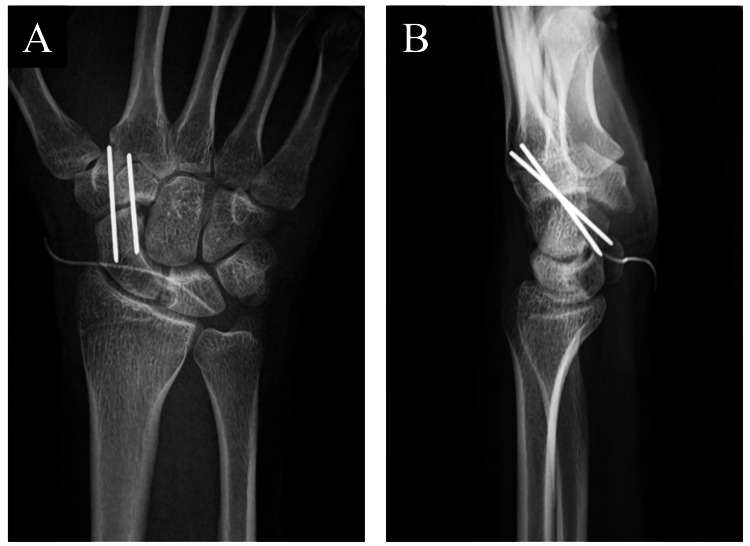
Postoperative radiographs of the left wrist. (A) Anteroposterior view. (B) Lateral view.
